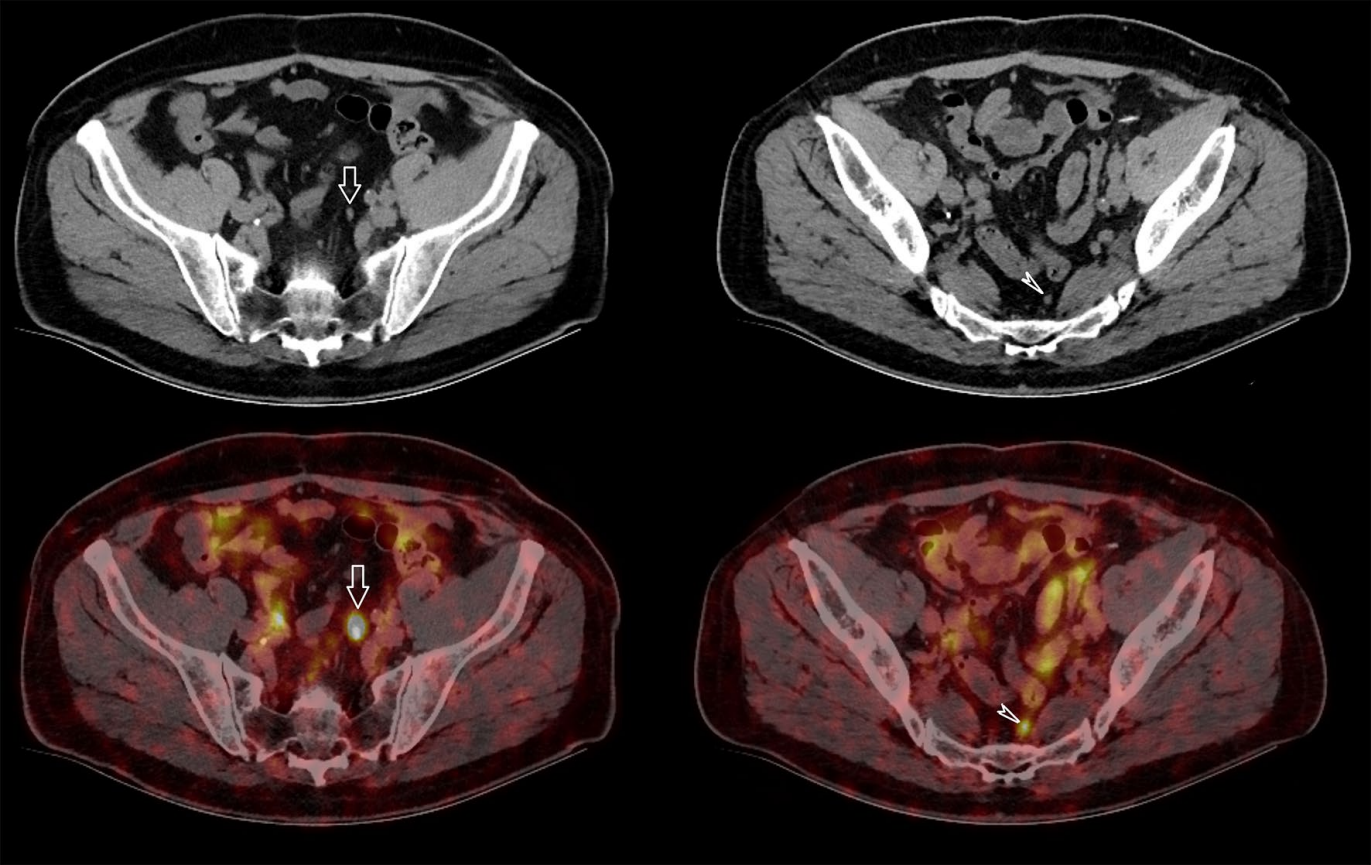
72-year-old man with biopsy proven PCa Gleason 4 + 3 pT2c and PSA of 6.7 ng/ml. Preoperative staging with imaging revealed two small lymph nodes in the pelvis, along the left common iliac artery (white arrow) and in the presacral fat (white arrowhead). On CT scan they both have a size of 5 mm which is below the morphological threshold to classify them as suspicious, but on ^68^Ga PSMA PET-CT they both showed highly avid tracer uptake, indicative of lymph node metastases. Pelvic lymph node dissection was performed and both lymph nodes proved to be malignant. Preoperative staging with ^68^Ga PSMA PET-CT thus appears to allow for more complete and accurate primary staging of PCa patients compared to standard routine imaging but its role in routine clinical practice is yet to be defined
